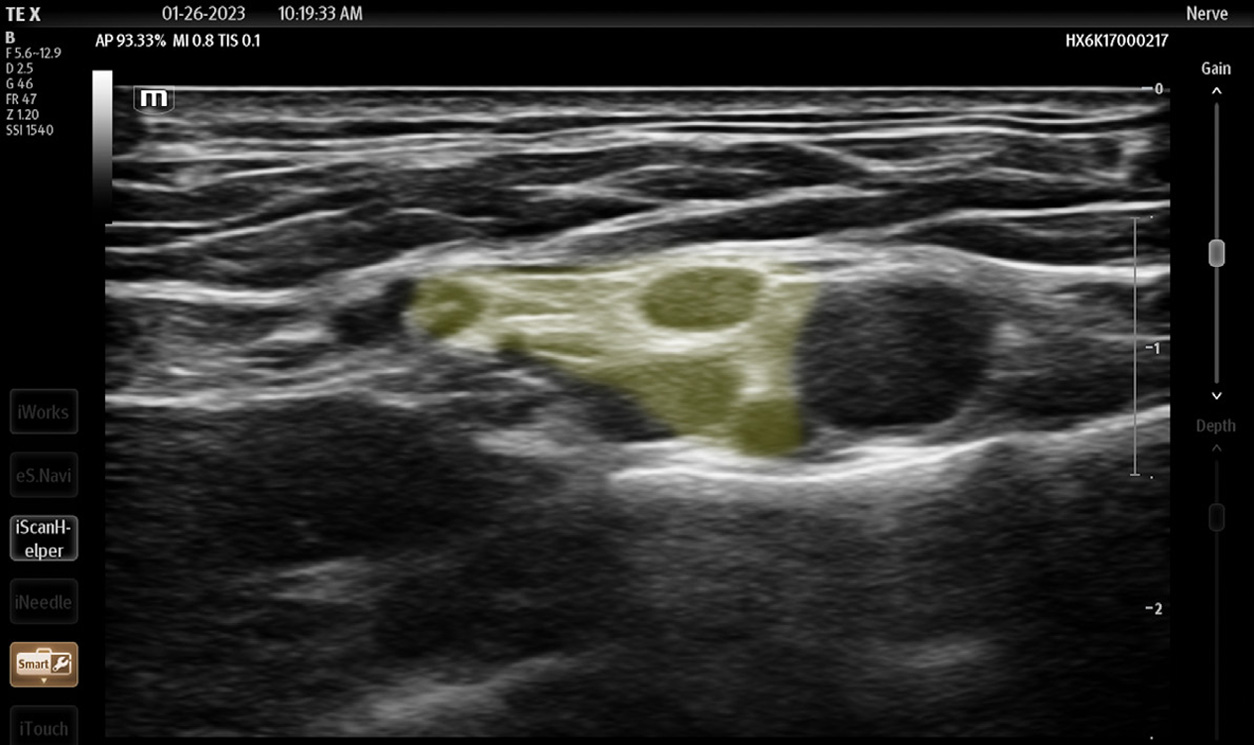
Ultrasound image of supraclavicular brachial plexus highlighted in yellow (Smart Nerve), Mindray TE X, Mindray North America, Image courtesy of Andrew Parrish.
